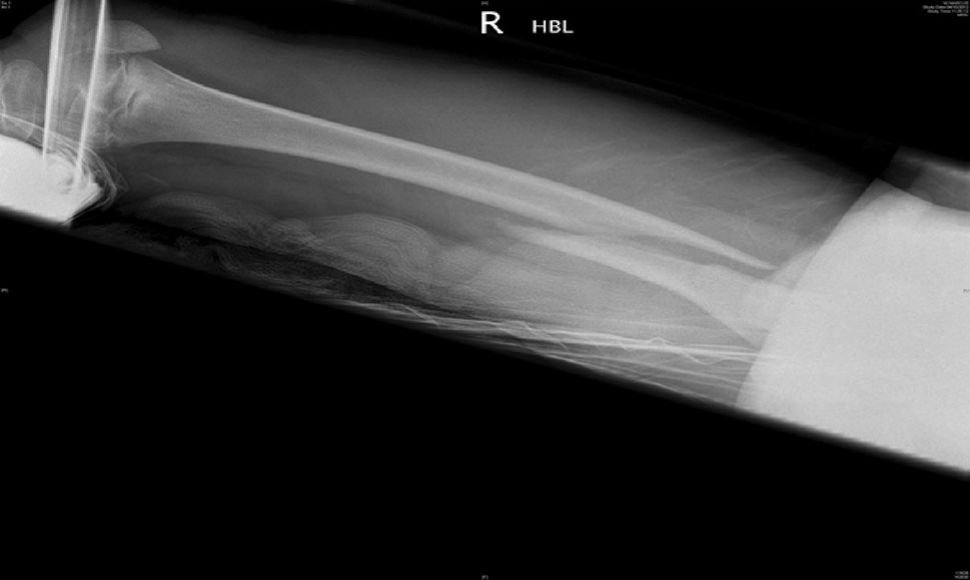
Lateral radiographs demonstrating a proximal 1/3 spiral femoral diaphyseal fracture in a 6-year-old child
